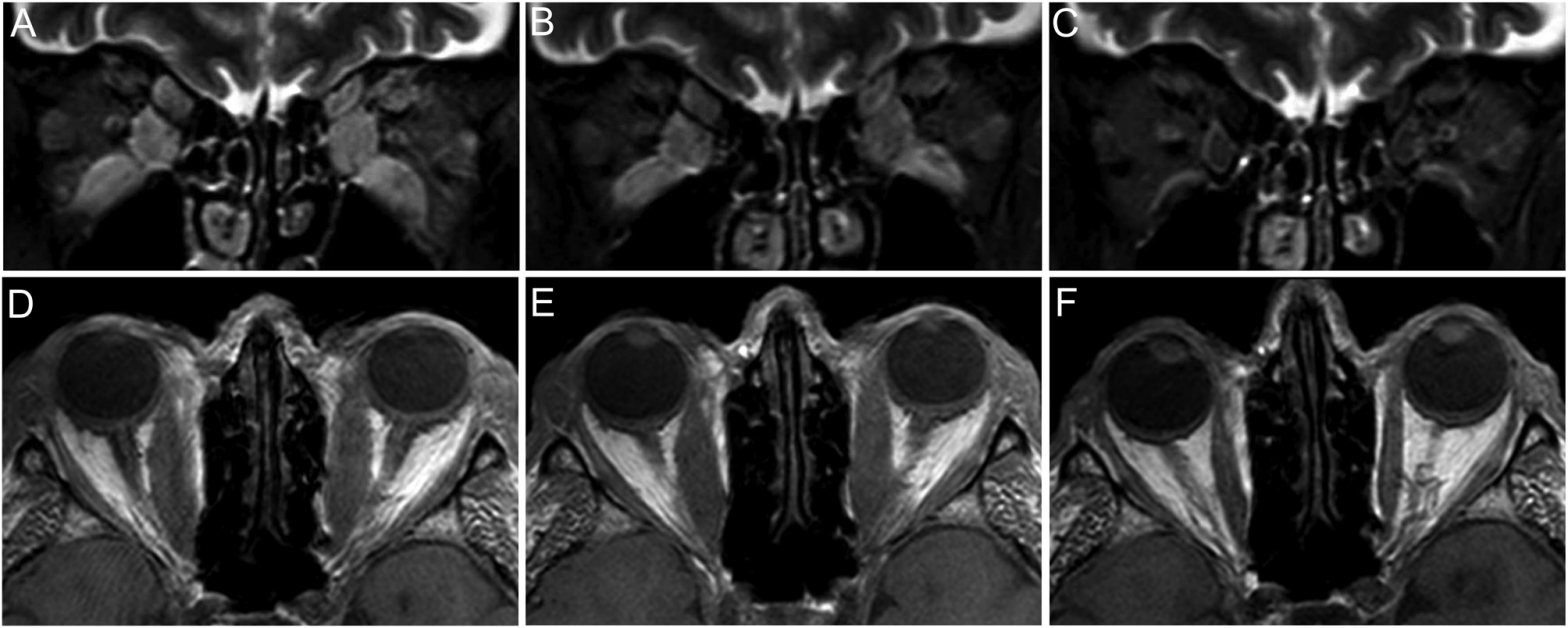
Treatment response. Short tau inversion recovery (STIR) coronal (A, B, and C) and T1-weighted axial (D, E, and F) MRI images. Higher signal intensities (bright areas) in the STIR images mean higher water content. (A and D) Day 1, before the administration of diuretics. (B and E) Day 4, after 3 days of diuretic treatment, before the first corticosteroid infusion. (C and F) Day 120, after diuretics and full course of corticosteroids combined with orbital irradiation.
